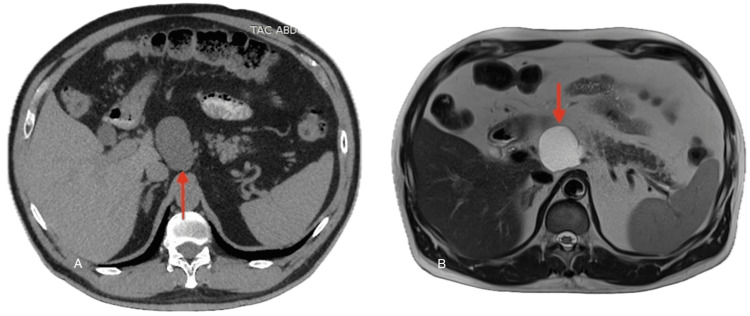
Cross-sectional images of the mesenteric cyst A: CT scan showing mesenteric cyst (red arrow); B: MRI showing mesenteric cyst (red arrow).
